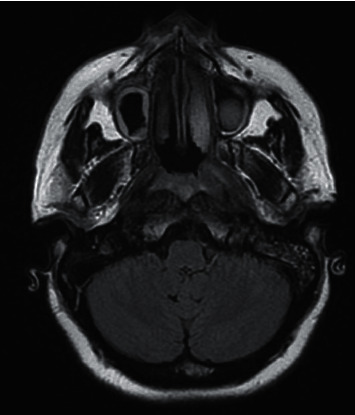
MRI brain axial view showing bilateral maxillary sinusitis and left mastoiditis.
